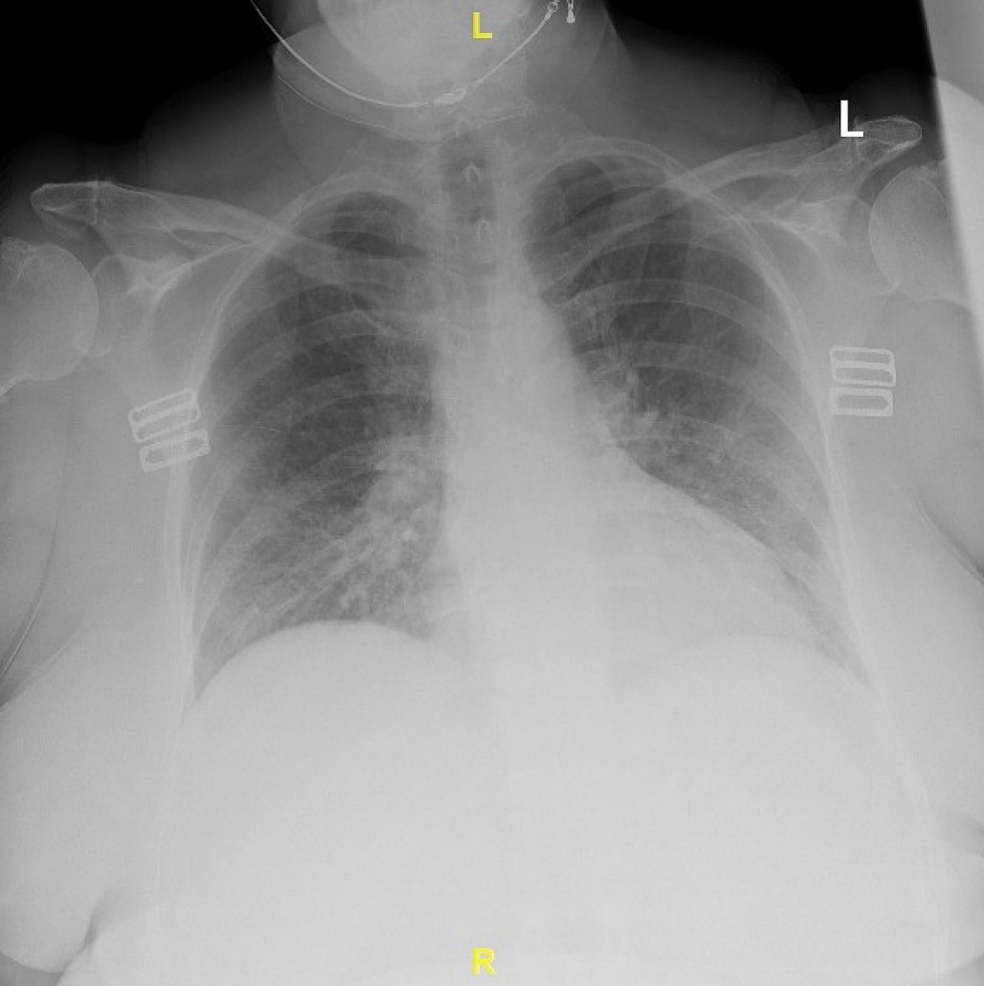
Chest X-ray The image shows mild pulmonary edema
